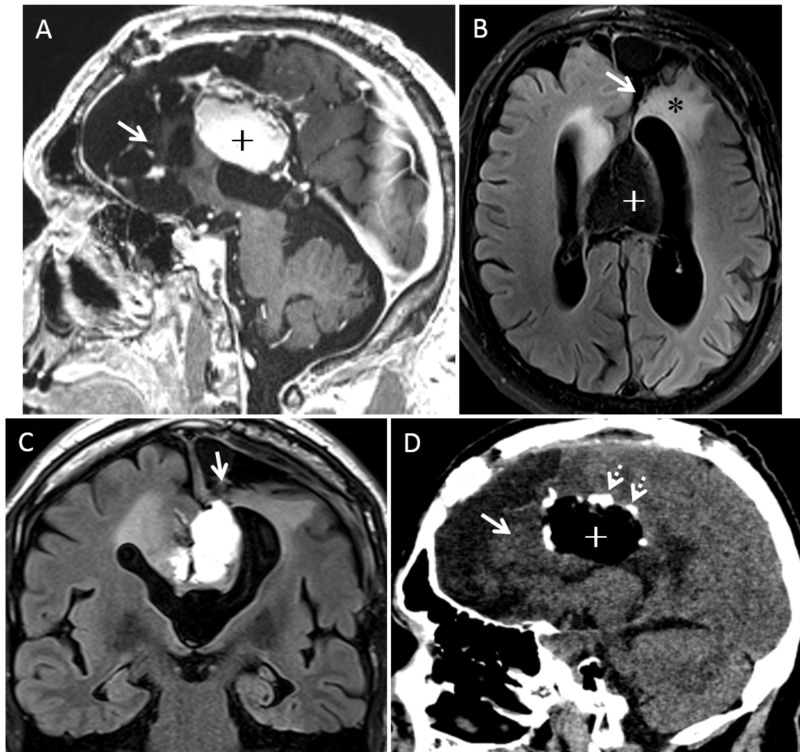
Post-operative images. A) Post contrast left parasagittal 3D T1 image shows resection of the large nodular calcified lesion in the left cingulate gyrus and medial frontal lobe (solid arrow). Pericallosal lipoma (+) is mostly intact. B and C) Axial fat-saturated and coronal nonfat-saturated FLAIR images show resection of the nodular calcified lesion and partial left frontal lobectomy (solid white arrow). Note the resolution of edema (*), mass effect and ex vacuo enlargement of the left frontal horn. Residual lipoma appears dark from fat saturation (+). D) Sagittal reformation of noncontrast CT demonstrates near total resection of the nodular calcified lesion in the left cingulate gyrus and frontal lobe. Residual calcifications are seen along the surface of the lipoma (+). CT: Computed tomography; FLAIR: Fluid-attenuated inversion recovery.
